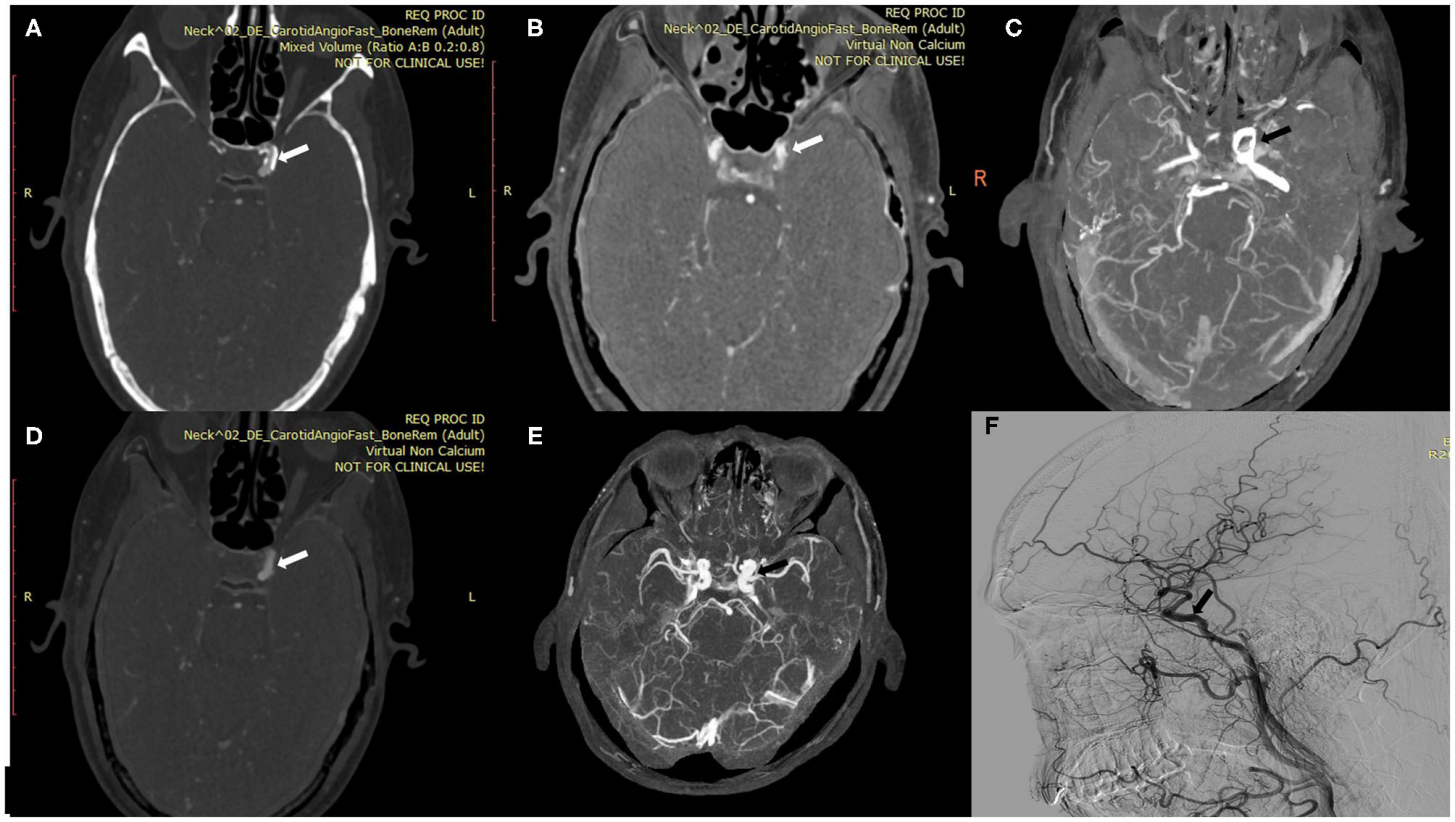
A 60-year-old man with calcified plaque on the left intracranial ICA. (A) Axial conventional CTA at the location of calcified plaque (arrow). (B,C) Axial VNCa 1 and MIP at the location of calcified carotid plaque (arrow). (D,E) Axial VNCa 2 and MIP at the location of calcified plaque (arrow). (F) DSA in sagittal projection indicated the no stenosis at the location of calcified plaque (arrow). The VNCa 1 still had residual calcification, and it tends to overcome the degree of calcified stenosis. Then VNCa 2 had good consistency with DSA.
